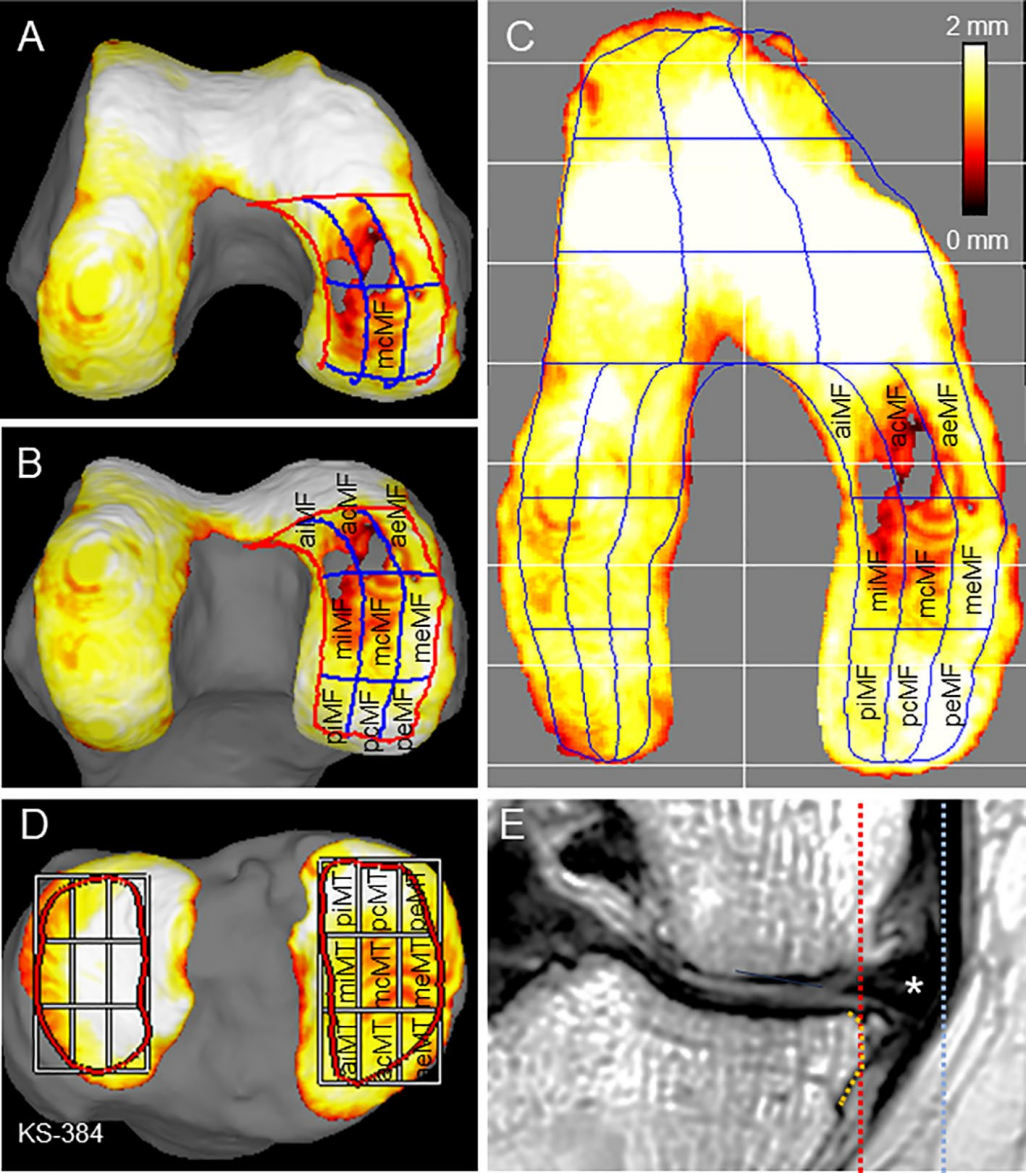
Measurement methods for subregions and medial meniscus extrusion (MME) in MRI. (A) Three-dimensional femoral cartilage viewed from below along the long axis of the femur. Cartilage thickness is shown with a color bar. (B) Three-dimensional femoral cartilage from a more posterior direction with all nine subregions visible. (C) Radially projected cartilage thickness mapping of the femoral cartilage and subregions of the medial femoral cartilage. The MF region is the combined area of the nine MF subregions. (D) Three-dimensional tibial cartilage and subregions of the medial tibial cartilage. The MT region is the combined area of the nine MT subregions. (E) Measurement of MME. A line along the original medial edge of the tibia (orange line) is drawn subjectively. A vertical line (red line) is drawn from the medial edge of the tibia, excluding the osteophytes, using a coronal plane MRI. A line (blue line) is then drawn from the outer edge of the medial meniscus (star). The distance between the red and blue lines is defined as the MME width. MF, medial femoral; aiMF, anterior internal MF; acMF, anterior central MF; aeMF, anterior external MF; miMF, middle internal MF; mcMF, middle central MF; meMF, middle external MF; piMF, posterior internal MF; pcMF, posterior central MF; and peMF, posterior external MF. MT, medial tibial; piMT, posterior internal MT; pcMT, posterior central MT; peMT, posterior external MT; miMT, middle internal MT; mcMT, middle central MT; meMT, middle external MT; aiMT, anterior internal MT; acMT, anterior central MT; and aeMT, anterior external MT.
